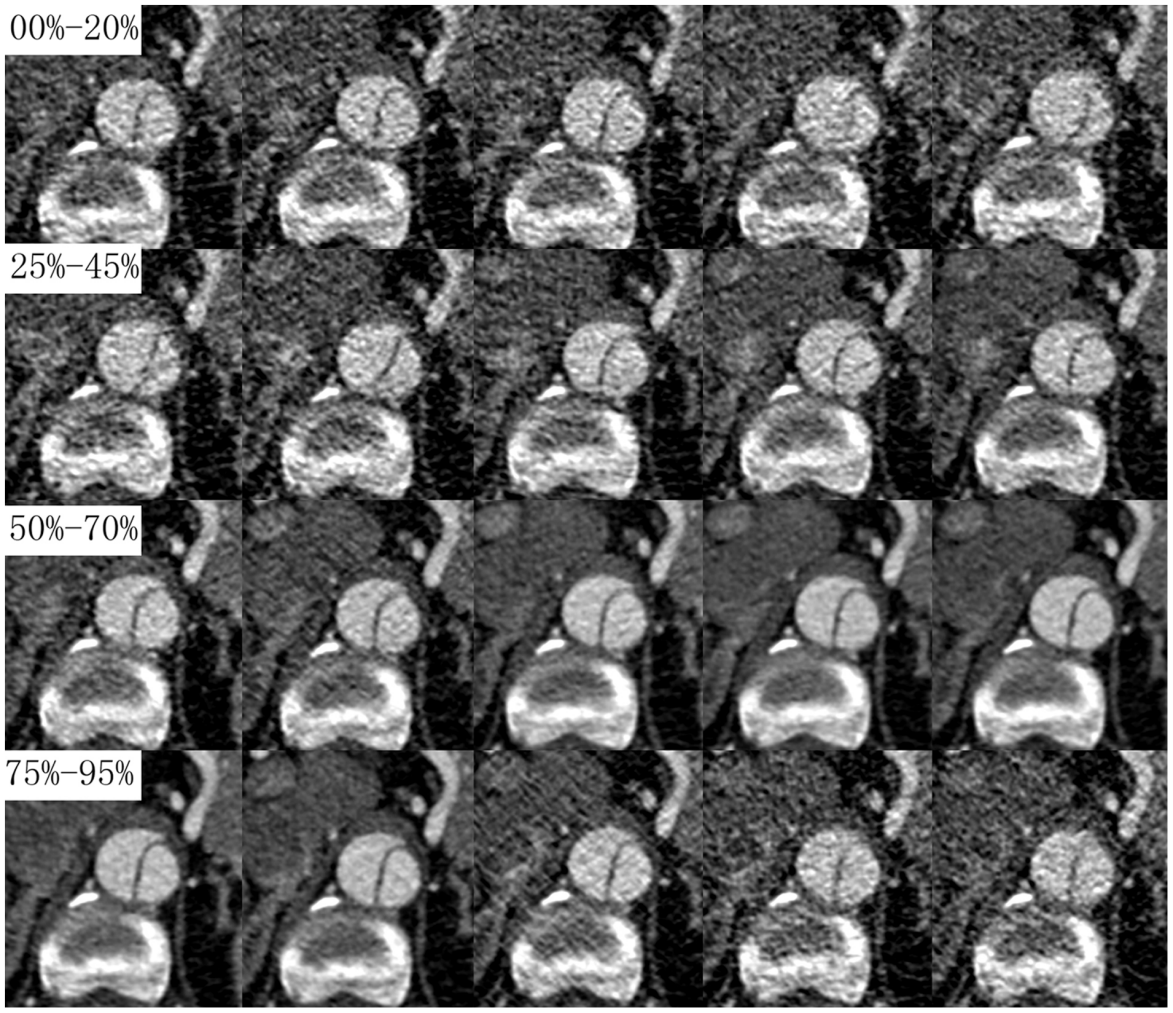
Transverse CT images of different phases at the same level. The intimal flap configuration, position and area of the true and false lumens were extremely variable during a cardiac cycle at the same level.
